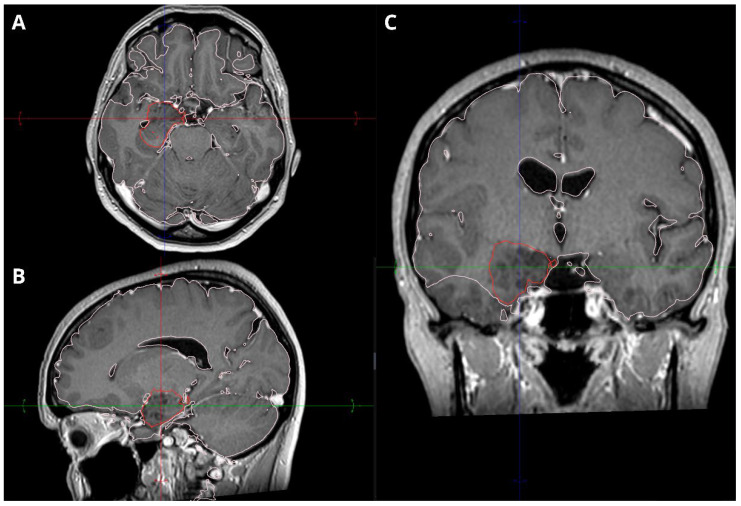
Primary dysembryoplastic neuroepithelial tumor (DNET) in the right hippocampus of a 47-year-old female at presentation. (A) Axial post-contrast T1-weighted MRI showing a well-demarcated, heterogeneous lesion in the right medial temporal lobe, with uncal herniation. (B) Sagittal post-contrast T1-weighted MRI revealing a medially located mass without significant brainstem deformation. (C) Coronal post-contrast T1-weighted MRI demonstrating the mass in contact with the ventricular wall, with no evidence of midline shift.
